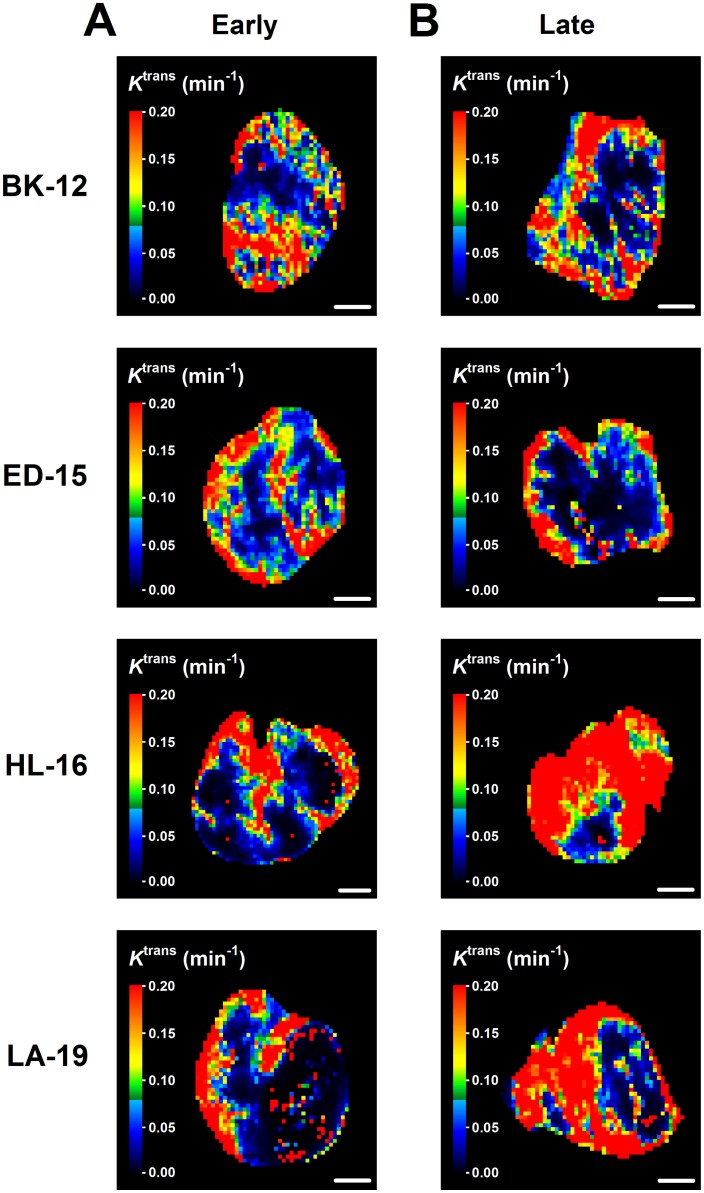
Dynamic contrast-enhanced magnetic resonance imaging-derived parametric images *K*^trans^ (volume transfer constant of Gd-DOTA) images of early (A) and late (B) generation BK-12, ED-15, HL-16, and LA-19 tumors. Scale bar: 2.0 mm.
